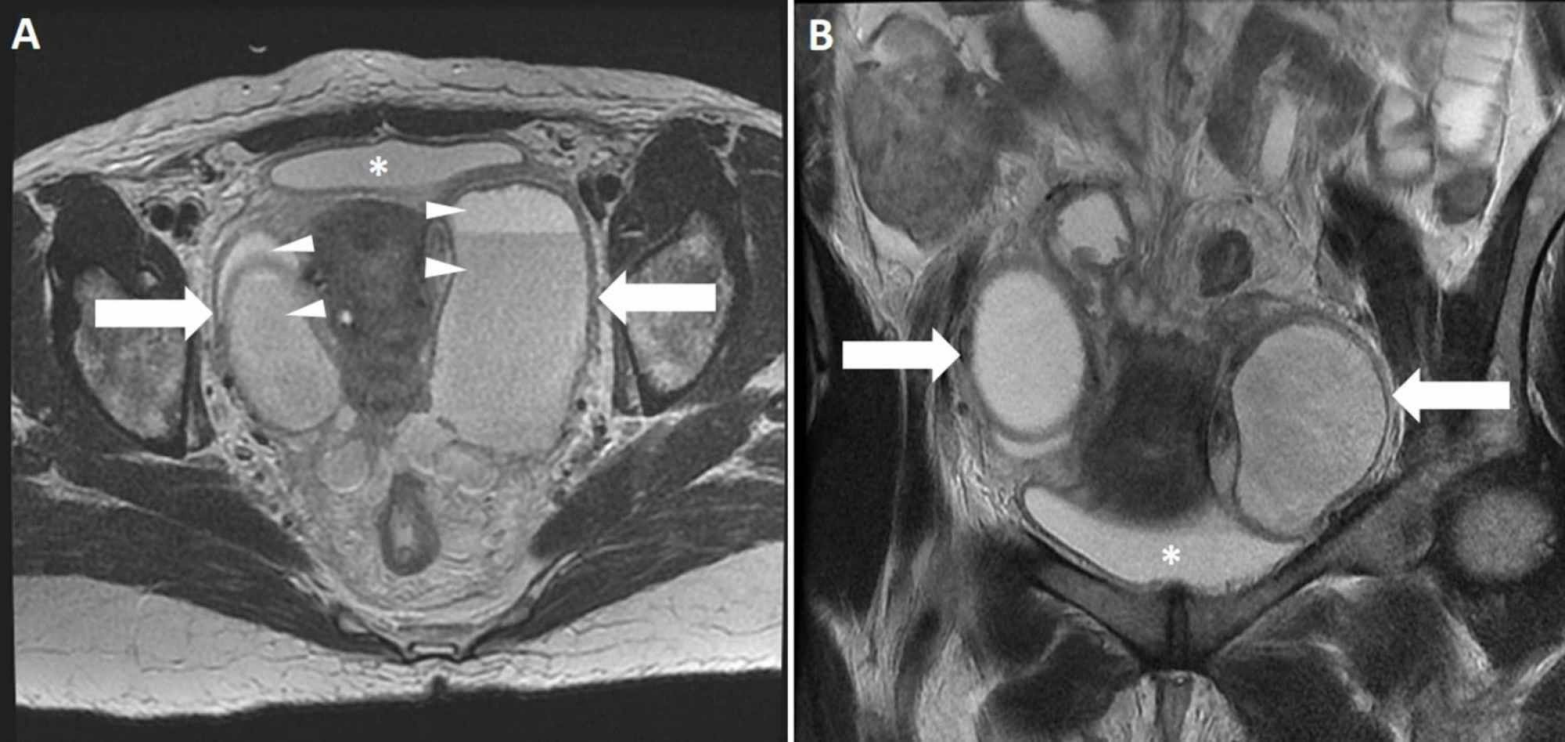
Axial (A) and coronal (B) T2-weighted MRI of the pelvis demonstrating bilateral adnexal cystic changes (arrows). Fluid-fluid levels (arrowheads) are seen in the axial view (A). The signal intensity (SI) of the fluid signal is similar to the SI of the fluid inside the bladder (asterisk). MRI, magnetic resonance imaging
